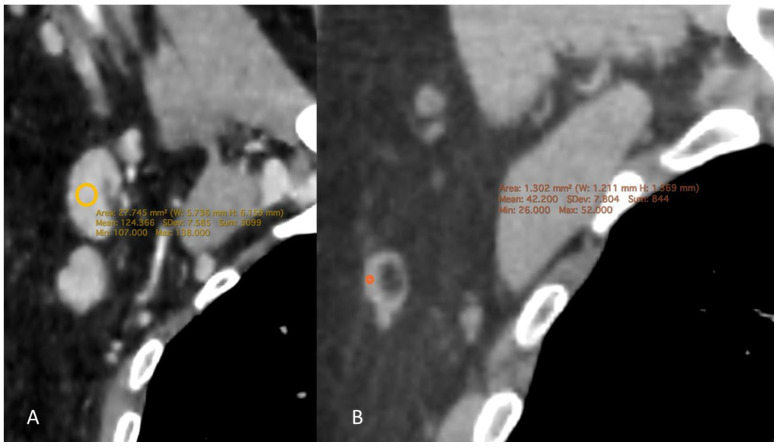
Contrast-enhanced chest CT coronal multiplanar reconstruction images demonstrating axillary lymph node cortical density in HIV-positive patients. (A) High plasma viral load; (B) Low plasma viral load.
